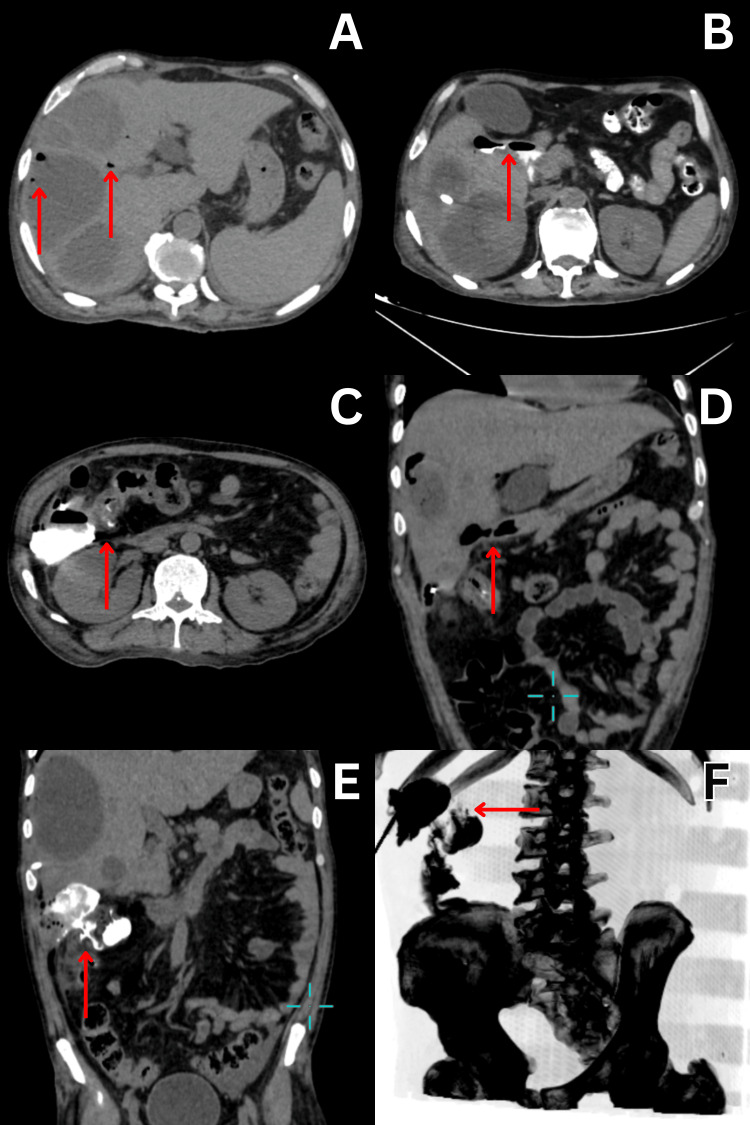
CT abdomen and fluoroscopy images (A) CT abdomen axial section showing multiple hepatic abscesses involving the right lobe of the liver with the presence of multiple air foci (arrow marks). (B) CT abdomen axial section showing abnormal fistulous communication (arrow mark) between the hepatic parenchyma and proximal part of the duodenum with contrast spillage. (C) CT abdomen axial section showing abnormal fistulous communication (arrow mark) between hepatic parenchyma and colon near hepatic flexure with contrast spillage. (D) CT abdomen coronal section showing the presence of an air-filled hepatoduodenal fistula (arrow mark) near the inferior surface of the liver. (E) CT abdomen coronal section showing the presence of a contrast-enhanced hepatocolic fistula (arrow mark) near the hepatic flexure. (F) CT abdomen 3D MIP B/W inverse image showing spillage of instilled contrast (arrow mark) through the hepatocolic fistula.
